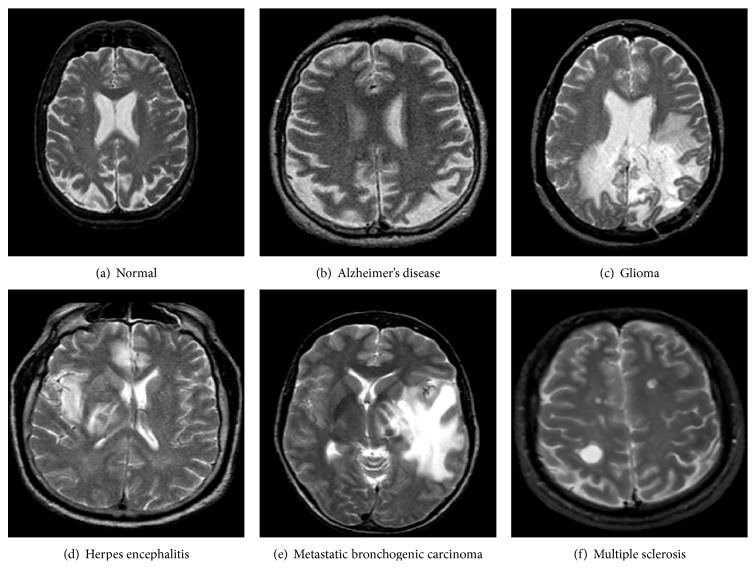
Examples of brain MR images.
